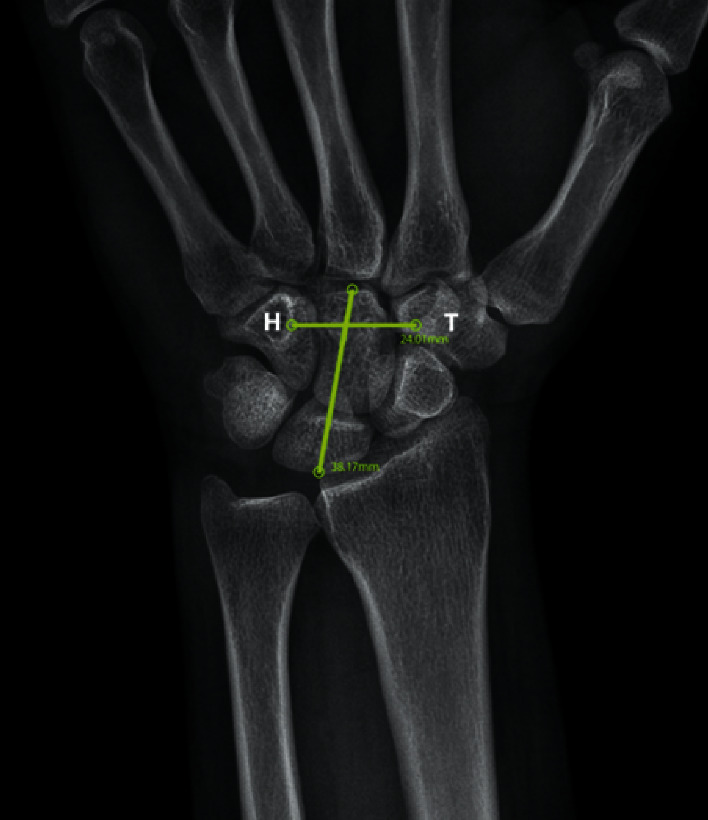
Decimal measurement of carpal tunnel dimensions by digital radiography. H, hamate; T, trapezium.
